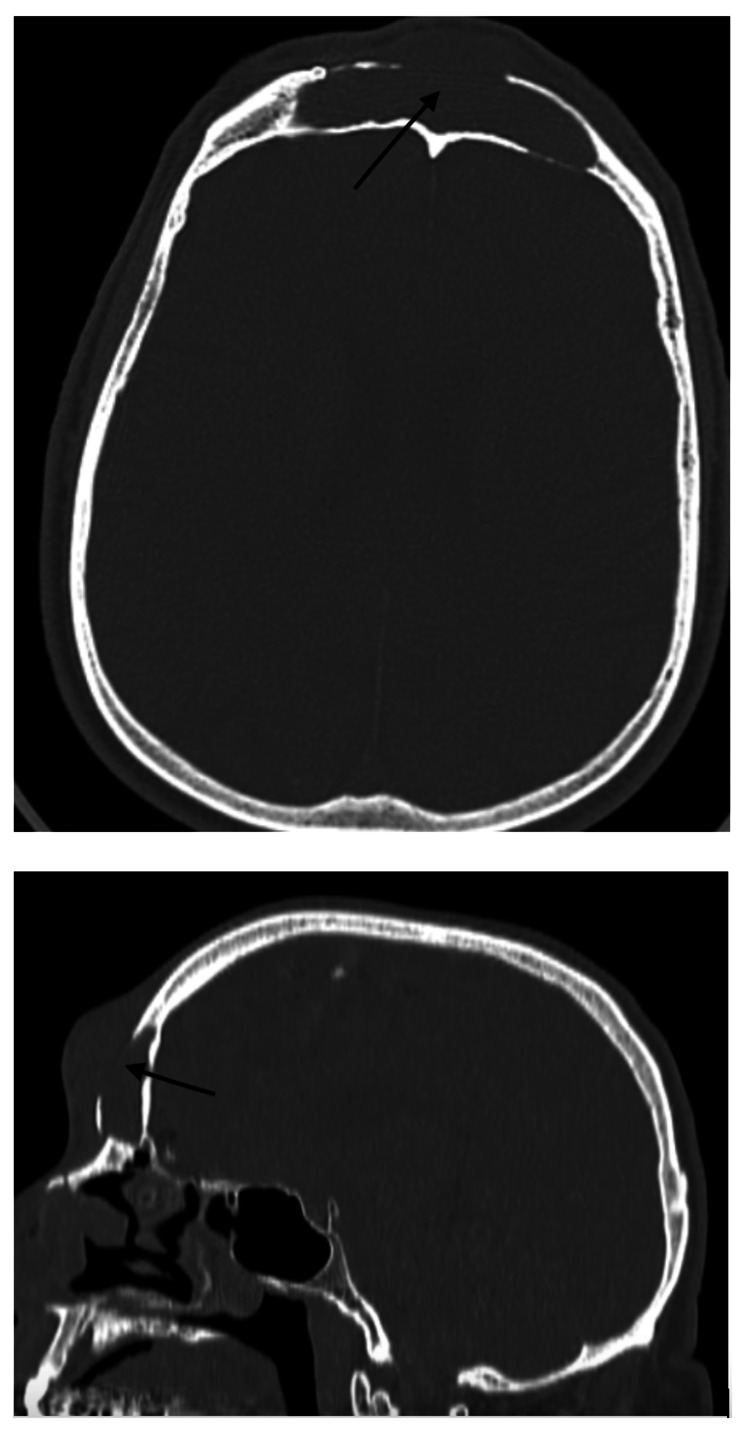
Axial and coronal CT scan showing mass via the destroyed anterior wall of the frontal sinus. CT: computed tomography
